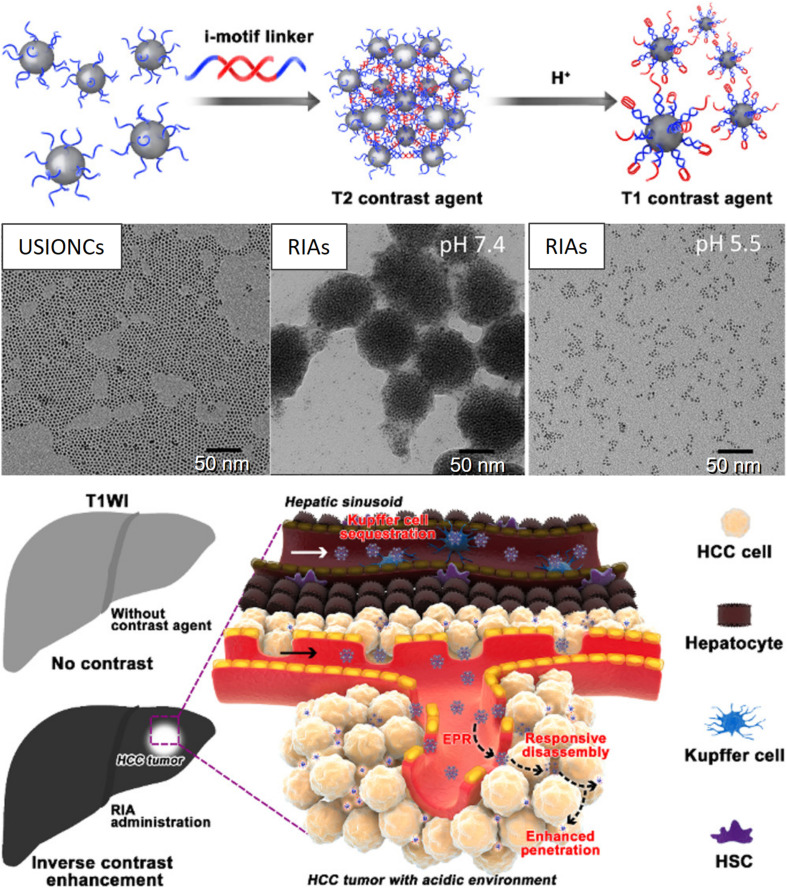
pH-responsive conversion of the MRI contrast agent confirmed via TEM images, and used for inverse contrast enhancement for the detection of small hepatocellular carcinomas (HCCs). Reproduced with permission from reference (Lu et al., 2018). Copyright 2018, American Chemical Society.
